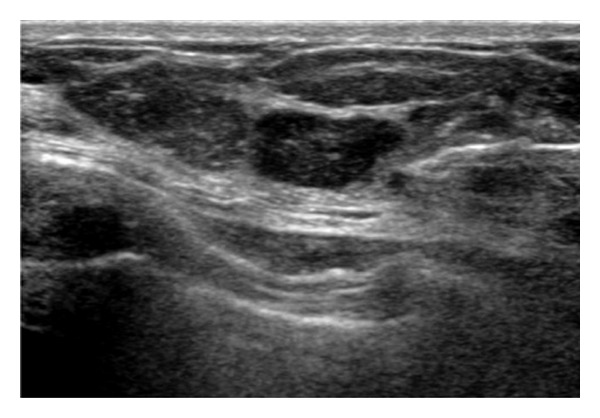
Ultrasound-guided biopsy of a lump from a patient with implant, allowing monitoring at all times the progress of the tip of the needle.
